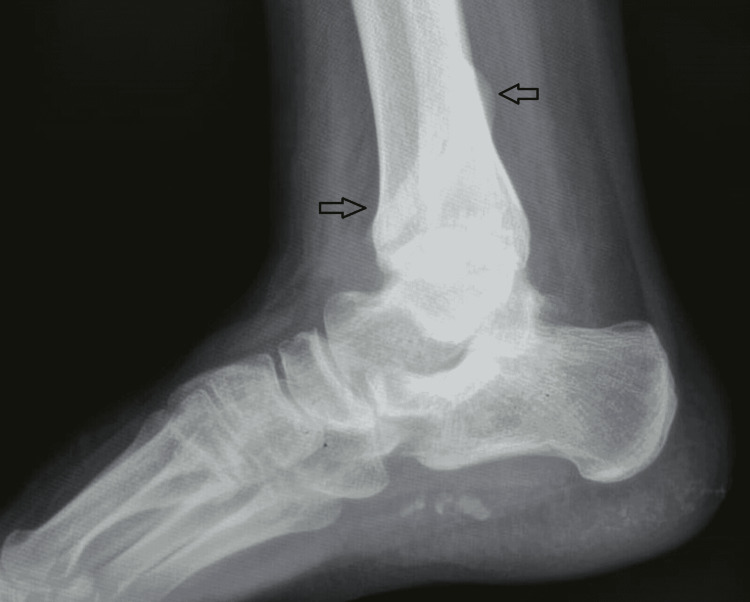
Preoperative radiograph lateral view showed lateral malleolus malunion
